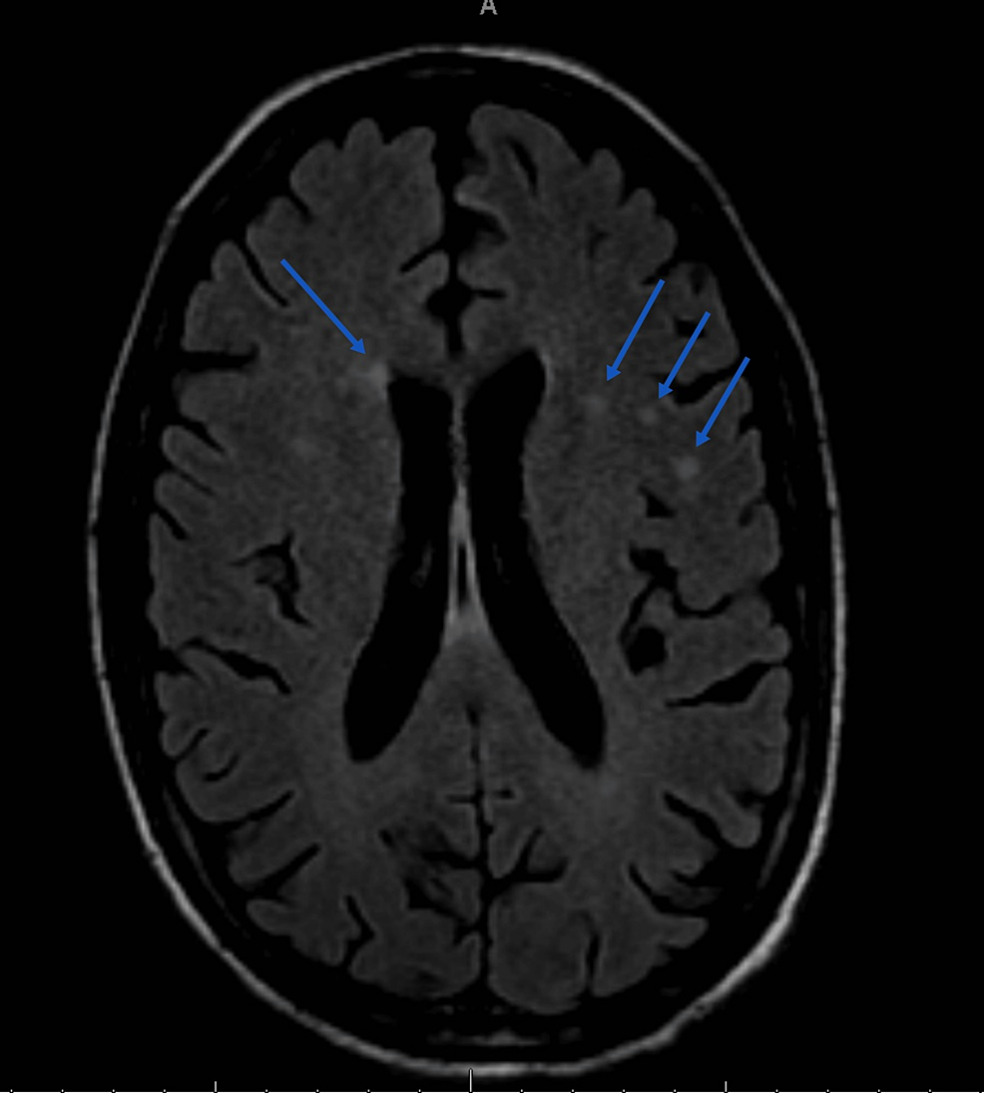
MRI brain with arrows pointing to restricted diffusion in the region of corona radiata and centrum semi-ovale. MRI: magnetic resonance imaging
